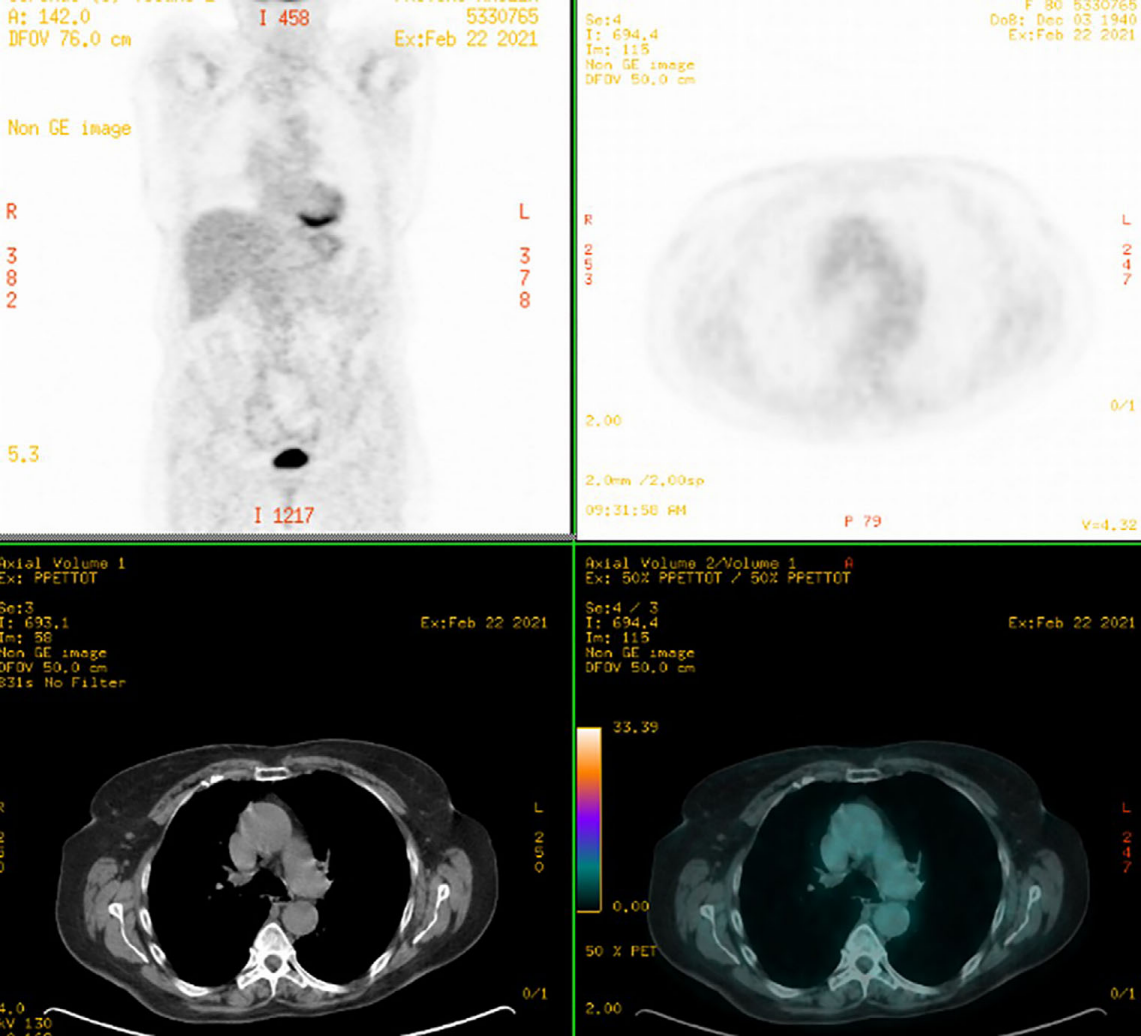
18F‐fluorodeoxyglucose (FDG) positron emission tomography (PET) performed for clinical staging did not show local or distant pathological uptake
